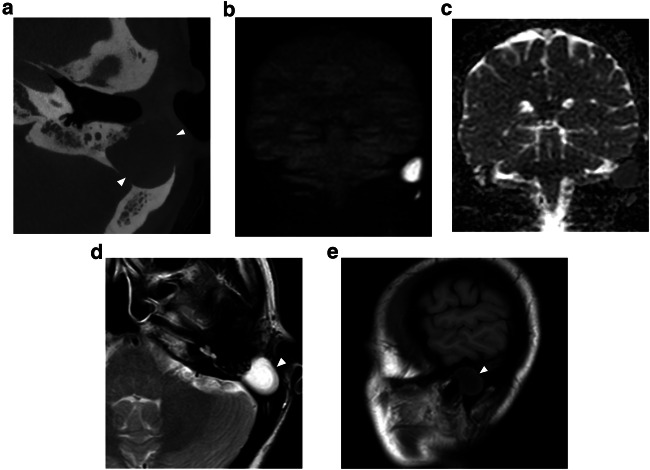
Thirty-eight-year-old women investigated for conductive hearing loss on the right with an incidentally found CC in the mastoid part of the left temporal bone (arrowheads in a, d, e). Note the convex edges, well-defined bone erosions and lack of inflammatory changes in the middle ear on the axial CBCT image (a). The MRI shows a typical high signal on DWI (b) and low signal on the ADC map (c), high signal intensity on T2-WI (d) and low signal intensity on T1-WI (e), compatible with a CC
